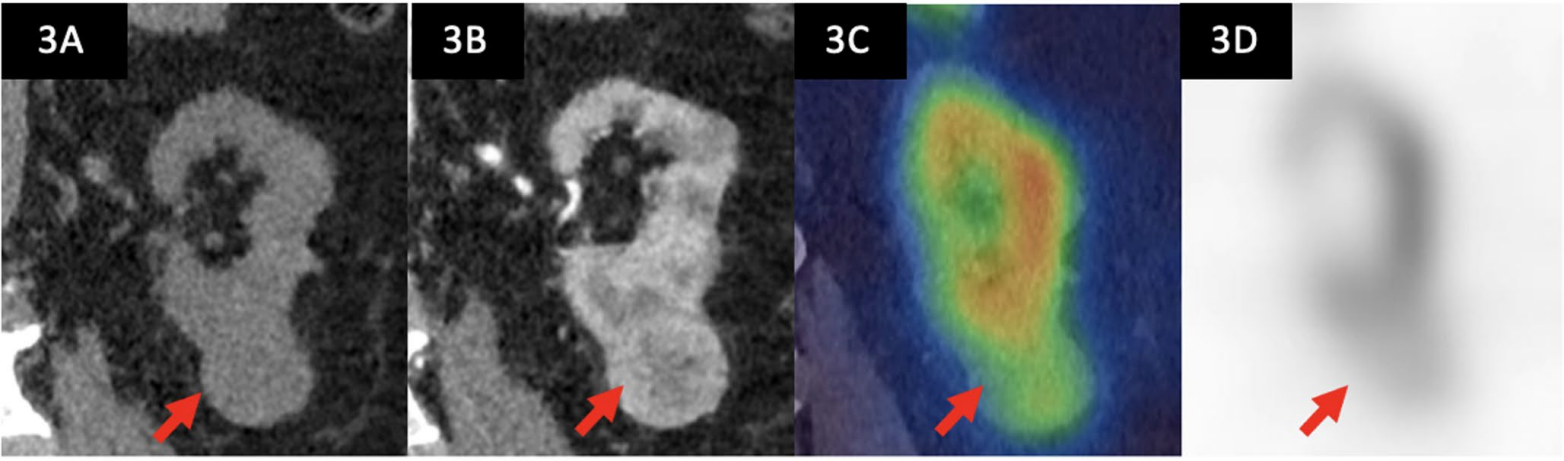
Diagnostic imaging of a 36mm left lower pole tumour indicated by the red arrow, diagnosed on biopsy as a renal oncocytoma. This tumour was interpreted as avid relative to the normal parenchyma of the ipsilateral kidney A) coronal non-contrast CT of the left kidney B) corresponding contrast-enhanced CT demonstrating a solid, enhancing, heterogenous tumour C) coronal [^99m^Tc]Tc-sestamibi SPECT-CT images shows radiotracer uptake, similar to that of the surrounding renal parenchyma D) corresponding coronal SPECT image shows [^99m^Tc]Tc-sestamibi uptake [Computed tomography = CT, Single photon emission computed tomography = SPECT]
